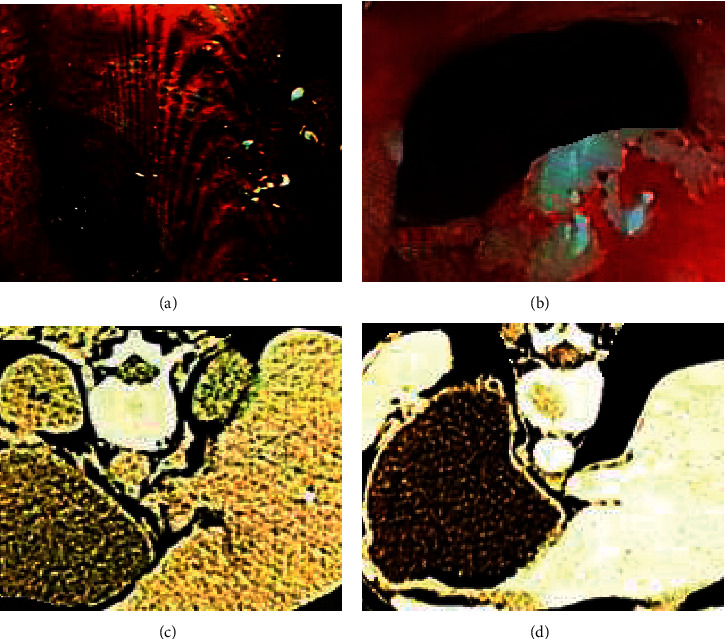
Imaging data of a 65-year-old man who visited the doctor because of epigastric discomfort for 2 weeks. (a) and (b) were the results of gastroscopy, while (c) and (d) were CT images.
